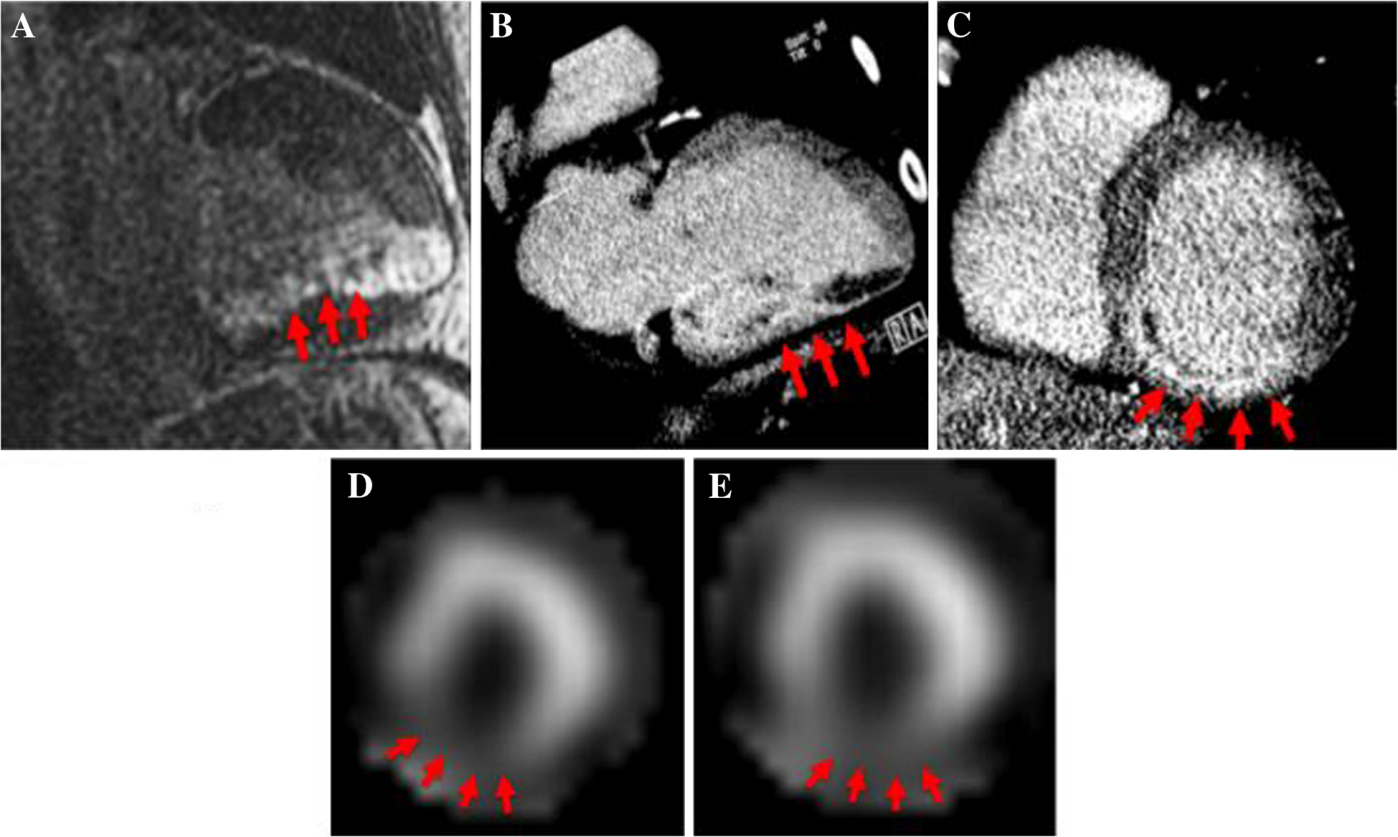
A 56-year-old male with hyperlipidemia presented with atypical chest pain. Cardiac MRI demonstrated scar (abnormal delayed enhancement--red arrows) in the entire inferior wall of the left ventricle as seen in two-chamber view (Panel A). CTA was performed to assess for previously unsuspected coronary disease; delayed images were performed confirming the same inferior wall delayed enhancement as seen in the two chamber and short-axis views (Panels B &C). The same patient underwent stress and rest Technitium-99m-Sestamibi SPECT- MPI (short axis images obtained at stress-Panel D and rest-Panel E), which corroborated the fixed defect in the inferior wall.
